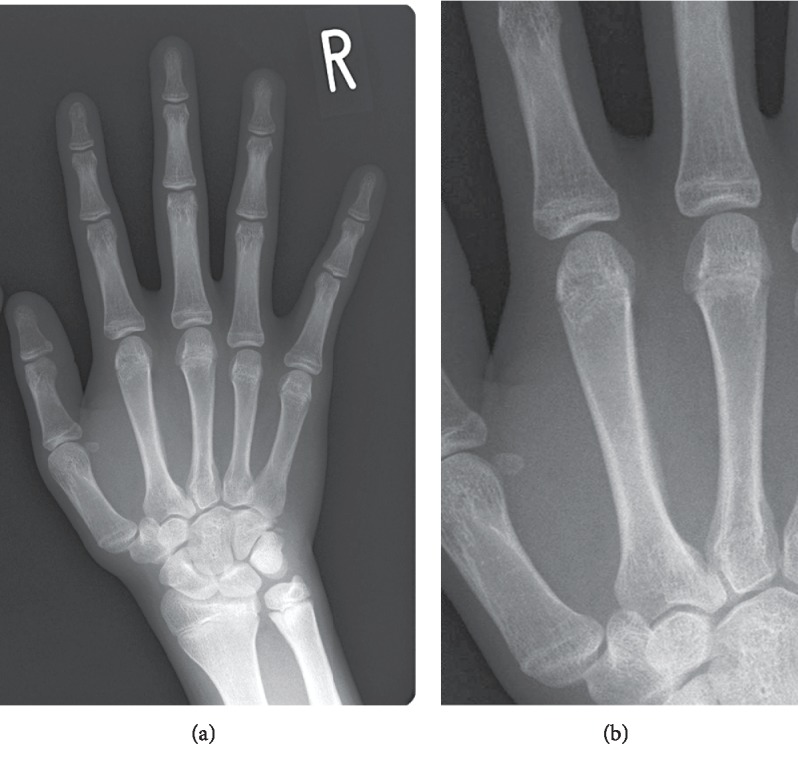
Right hand radiograph showing a periosteal reaction at the ulnar side in the shaft of the second metacarpal.
